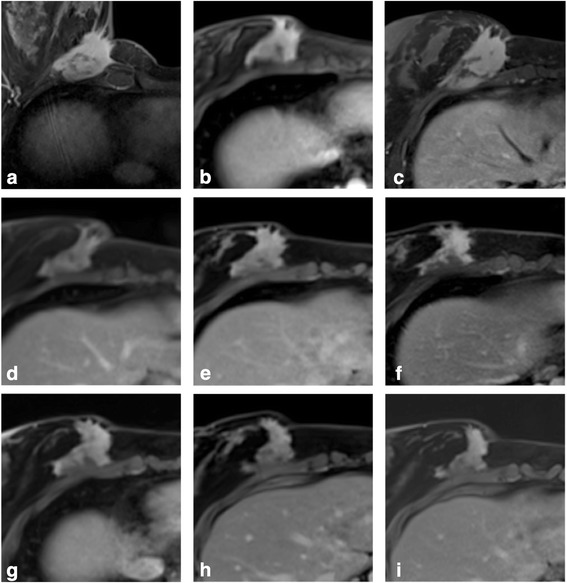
Tumor evolution on MRI. a–c MRIs during tamoxifen + arthrocine association (tumoral volume respectively 27.4, 27.1, and 30.4 cm^3^). d–f MRIs during sorafenib (tumoral volume respectively 24.7, 26.5, and 25.6 cm^3^). g MRI during IFN (tumoral volume 26.4 cm^3^). h, i MRIs during sunitinib (tumoral volumes respectively 15.3 and 13.2 cm^3^)
